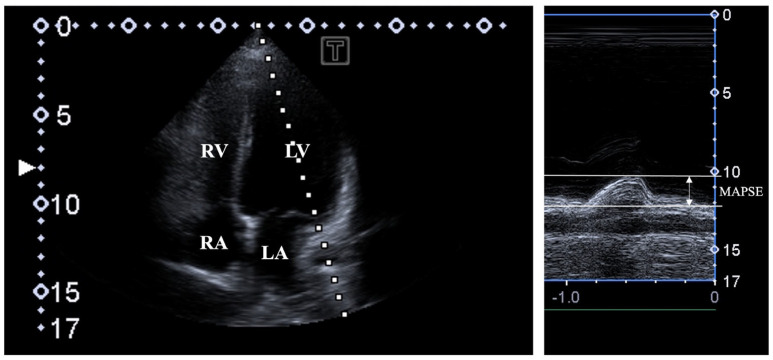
Assessment of mitral annular plane systolic excursion (MAPSE) by M-mode echocardiography in apical four-chamber view. Abbreviations: LA = left atrium; LV = left ventricle; RA = right atrium; RV = right ventricle; MAPSE = mitral annular plane systolic excursion.
